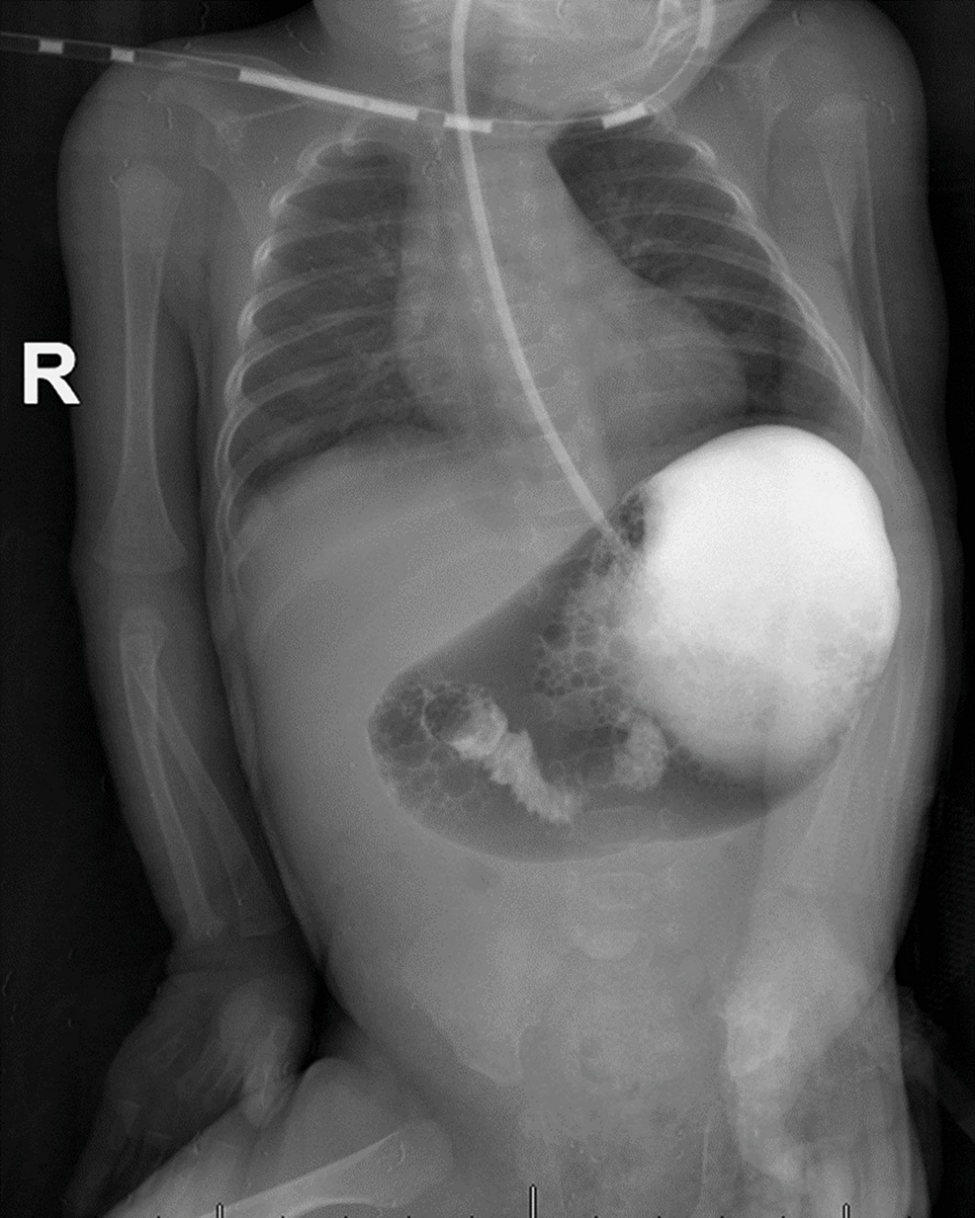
X-ray imaging with contrast medium revealing complete bowel obstruction, highlighting the severity of the condition
